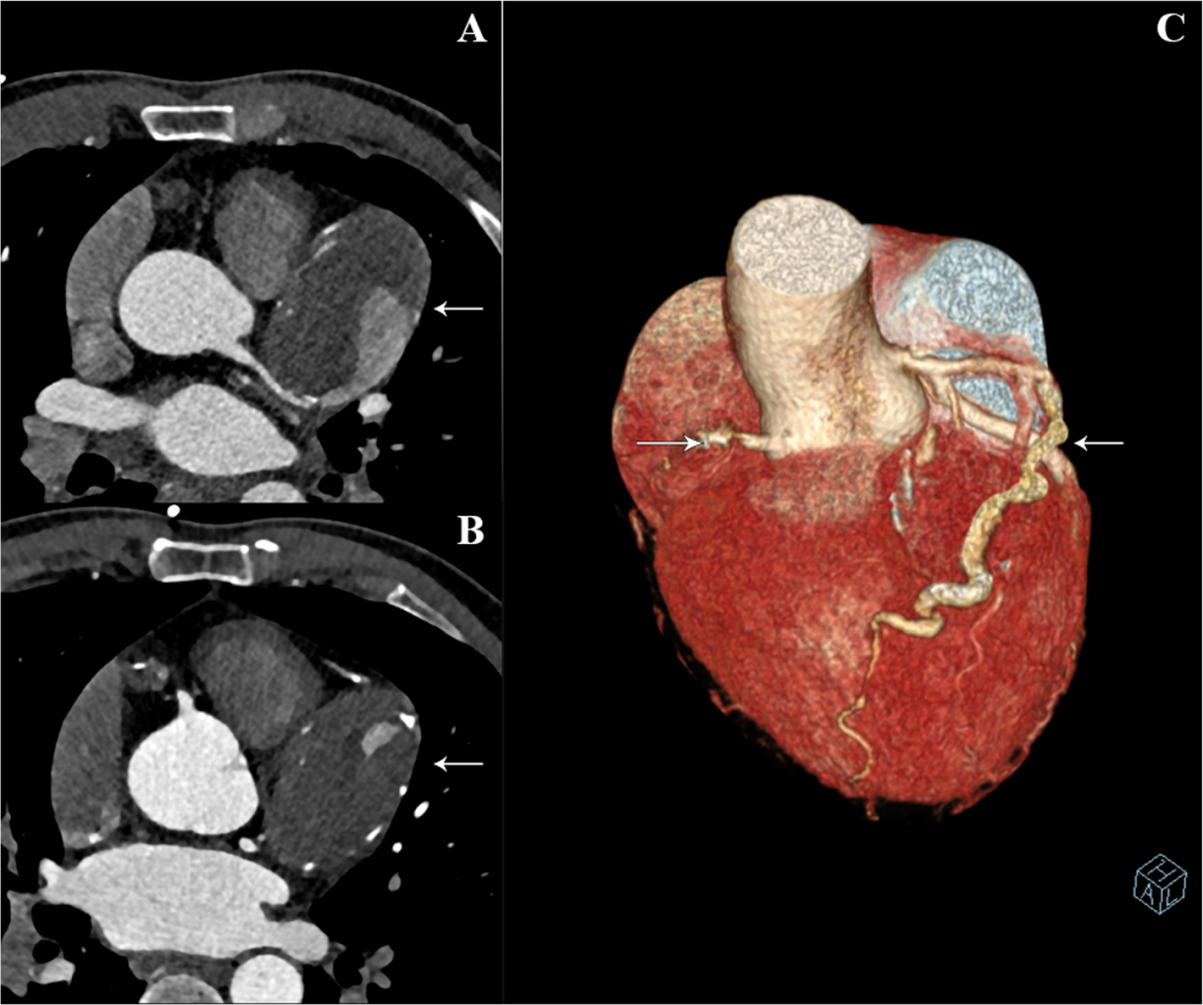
Dual-source computed tomography showing the giant aneurysm and the affected vessel
